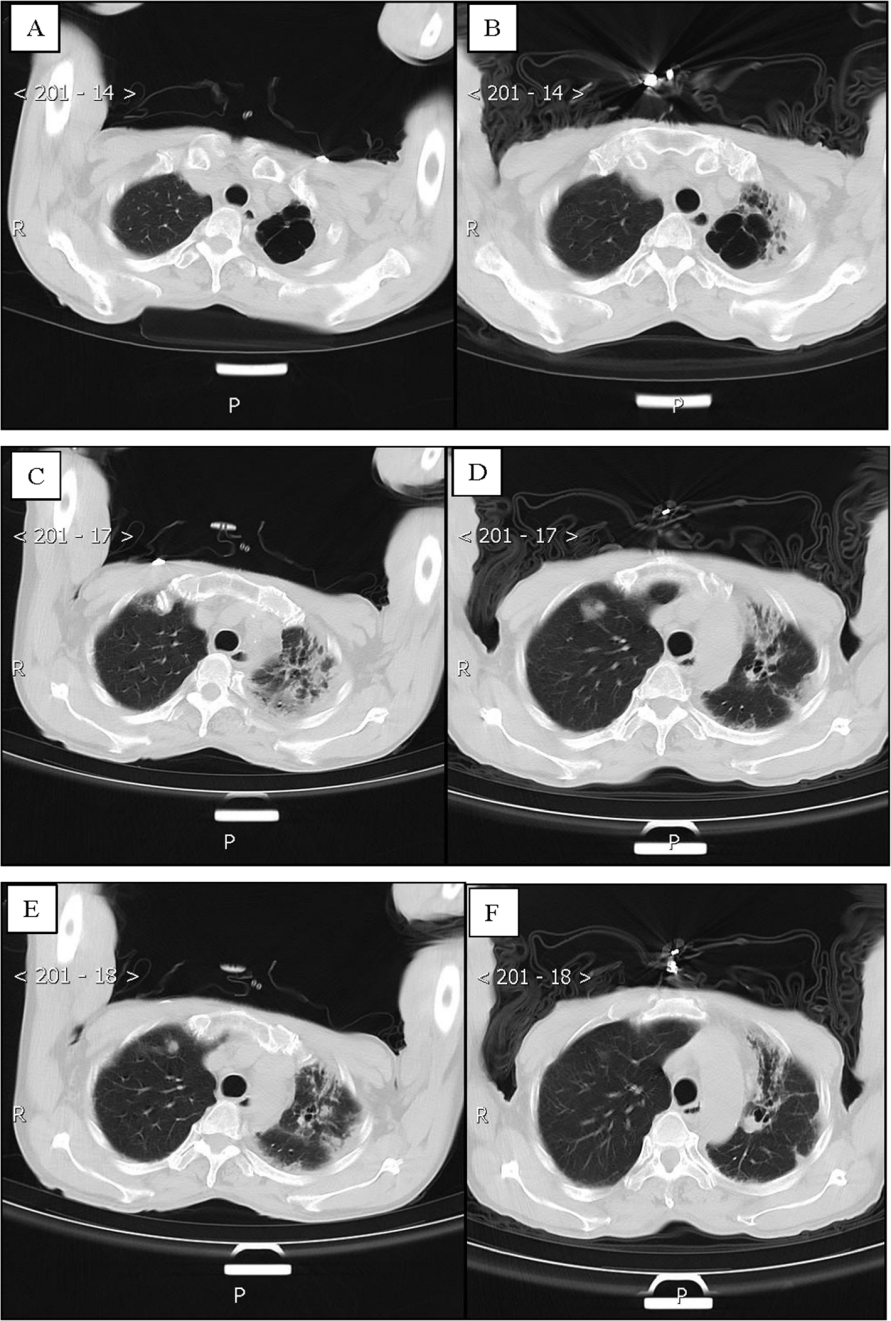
Chest CT images of A 75-year-old man with COVID-19 infection and bronchiectasis, obsolete tuberculosis. a, c, e Transverse chest CT images revealed extensive patchy exuding shadows and fibre stripe in left lung, and damage changes of upper lobe or left lung on admission. b, d, f Transverse chest CT images showed patchy exuding shadows mostly was absorbed in left lung on discharge
